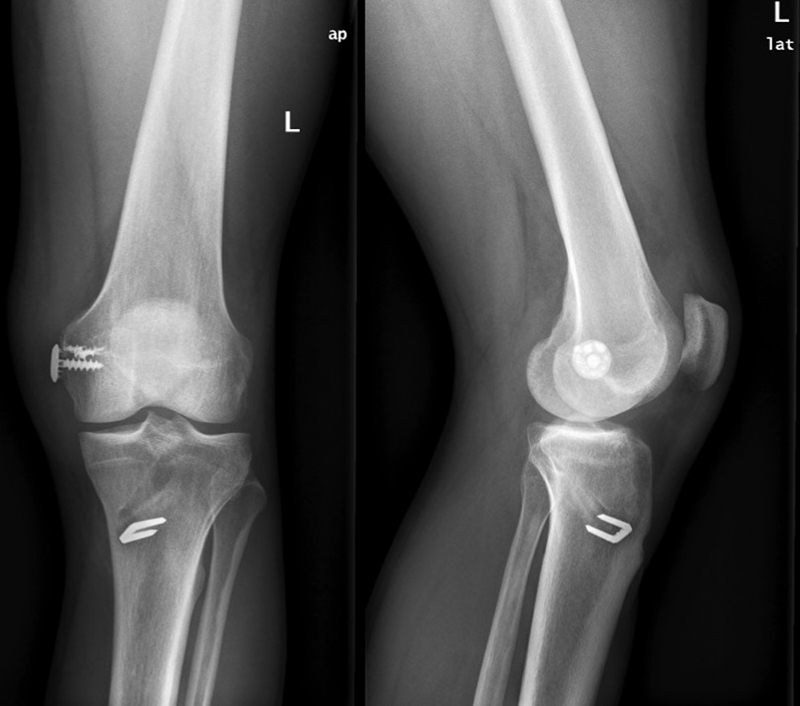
postoperative X-rays at the last follow-up.
